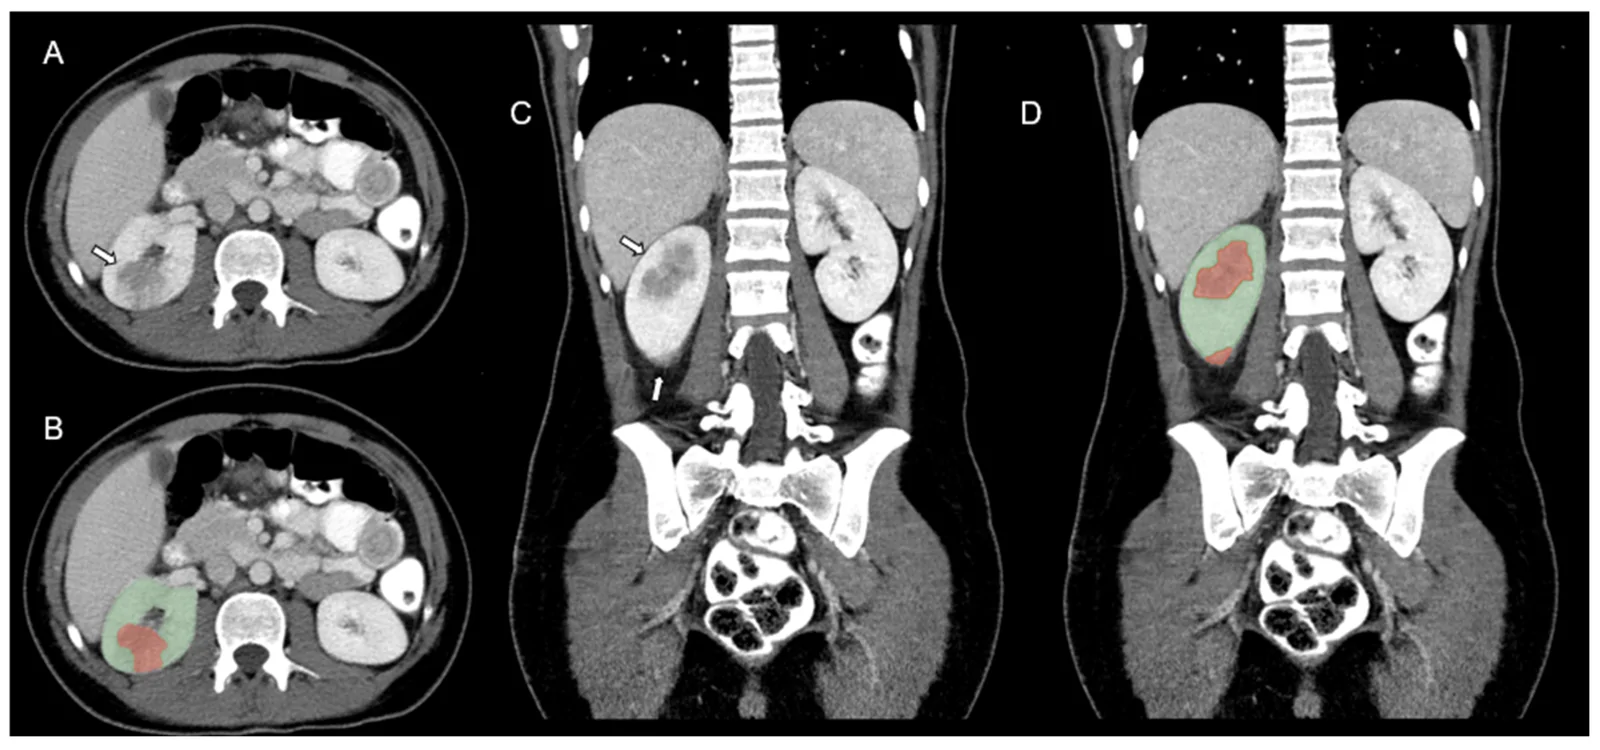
Contrast-enhanced computed tomography (CT) imaging identified multifocal perfusion deficits in the right kidney of a female patient, indicated by white arrows in the axial (A) and coronal (C) planes. Panels (B,D) show the corresponding segmentation overlays used to quantify the total volume of the affected kidney and the perfusion deficit, with the kidney segmentation shown in green and the perfusion deficit shown in red. The perfusion defect volume was 20.6 cm^3^, accounting for 12.3% of the total affected kidney volume of 168.0 cm^3^. The patient had a C-reactive protein (CRP) level of 202 mg/L and a white blood cell count (WBC) of 20.17 × 10^9^/L.
